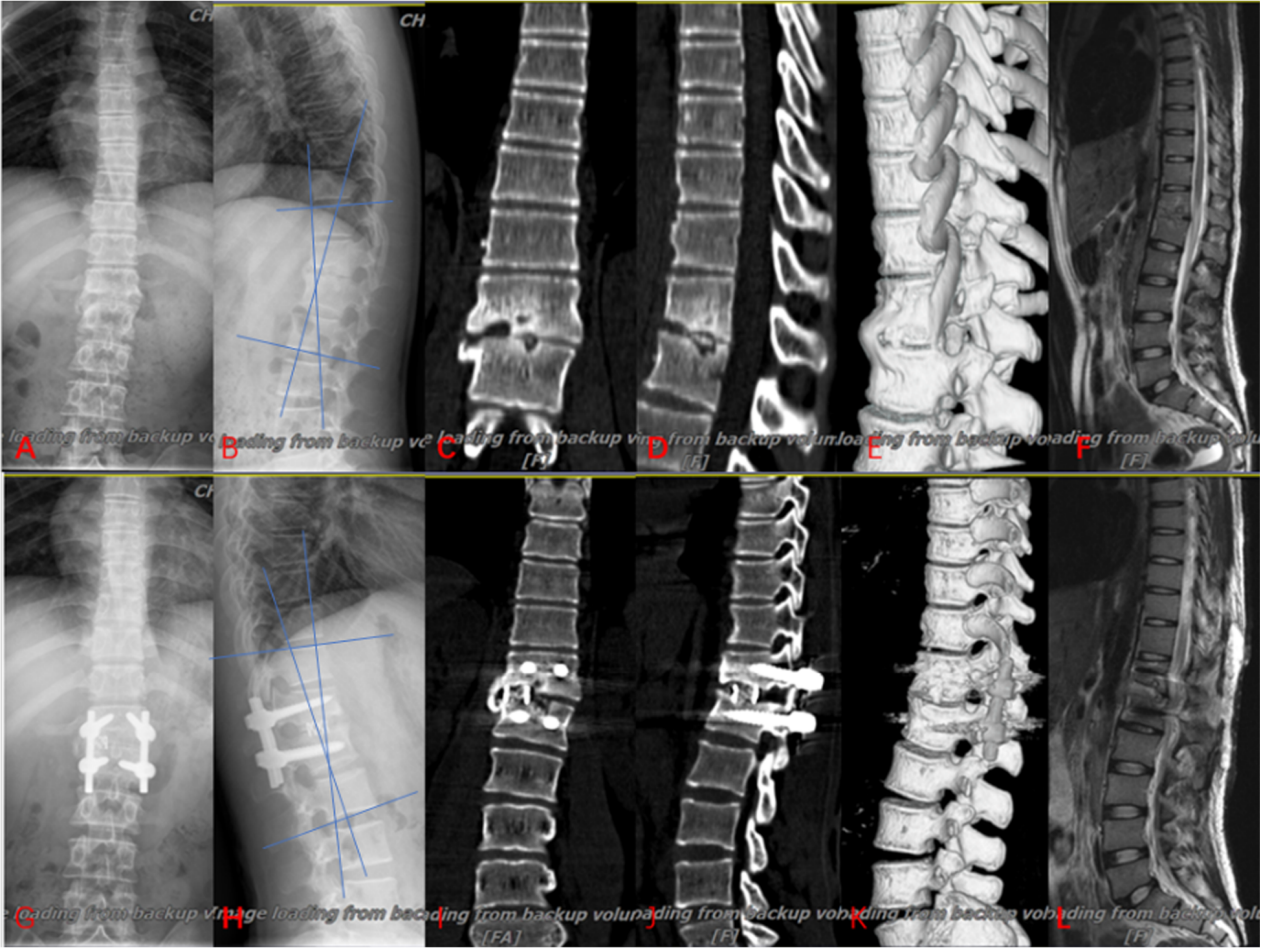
The graph showed a case underwent posterior debridement, decompression, bone grafting and internal fixation instrumentation. 18-year-old man with thoracolumbar junction (T12-L1) TB (a, b) preoperative anteroposterior and lateral X-rays; c, d CT preoperative; e 3D reconstruction of CT preoperative; f preoperative MRI; g, h X-ray at 1-month postoperative; i, j CT at 3-month postoperative; k 3D reconstruction of CT at 12-month postoperative; l MRI at 12-month postoperative
